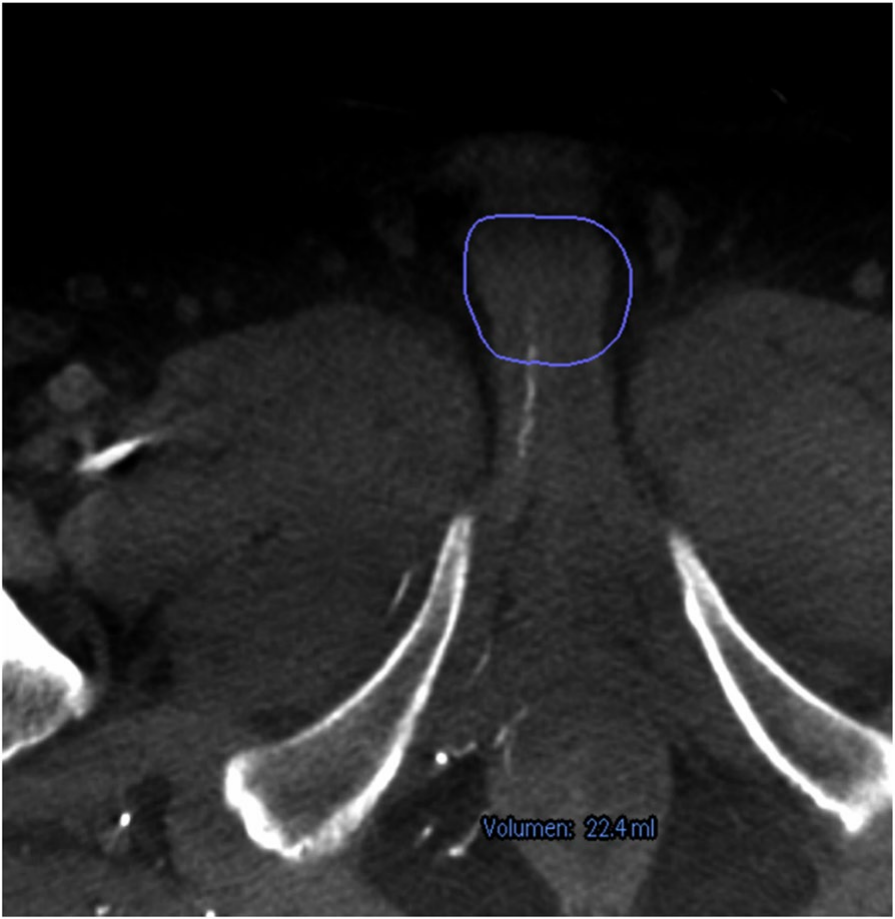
After performing a cone-beam computed tomography (CBCT) the EmboGuide® software (Philips) is used to calculate a road map. As a first step the root of the penis is marked as navigation target (blue circle). Shown are the images of a 63-year-old patient with arteriogenic erectile dysfunction
